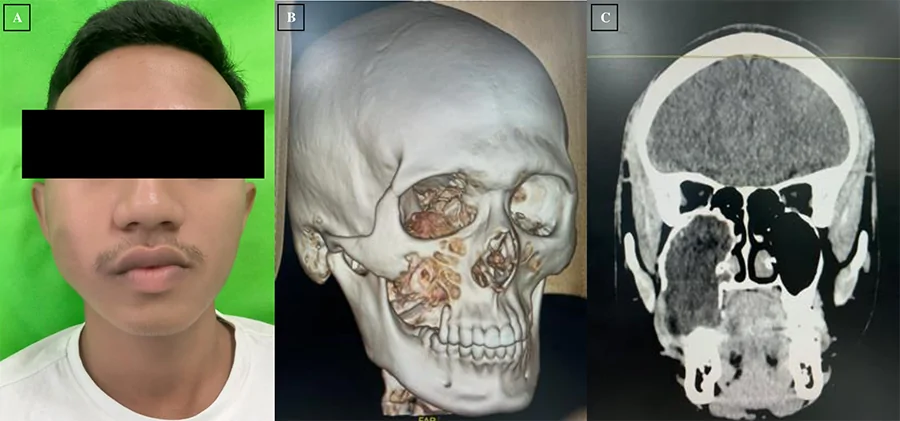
(A) Extraoral examination showing facial asymmetry; (B-C) Three-dimensional coronal CT scan view.
